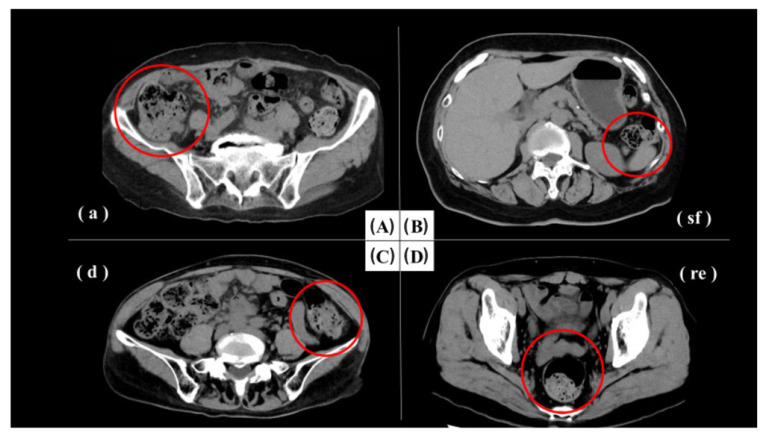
Representative CT imaging for constipation diagnosis. (A) CT image was used to rate the stool volume as 4 and the gas volume as 2 (red circle). (a) Ascending colon. (B) CT image was used to rate the stool volume as 1 and the gas volume as 2 (red circle). (sf), splenic flexure. (C) CT image was used to rate the stool volume as 3 and the gas volume as 2 (red circle). (d) Descending colon. (D) CT image was used to rate the stool volume as 3 and the gas volume as 3 (red circle). (re), rectum. Reprinted with the permission of [261].
